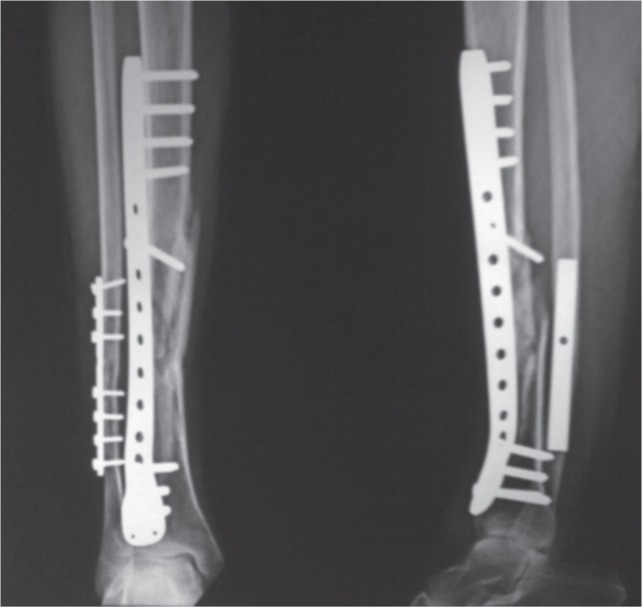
Anteroposterior and Lateral radiograph showing union of fracture of tibia and fibula of same patient shown in Figure 2 and 3.
